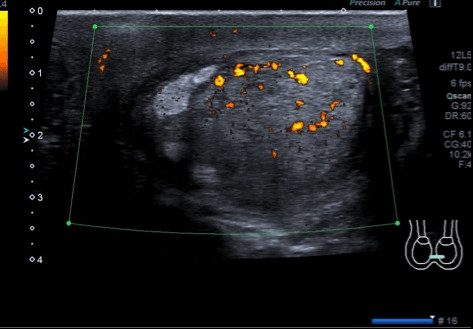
Ultrasonography and colour Doppler examination of the schwannoma. The lesion appeared inhomogeneous, was partially hypoechogenic, and had poor hypervascularisation.
